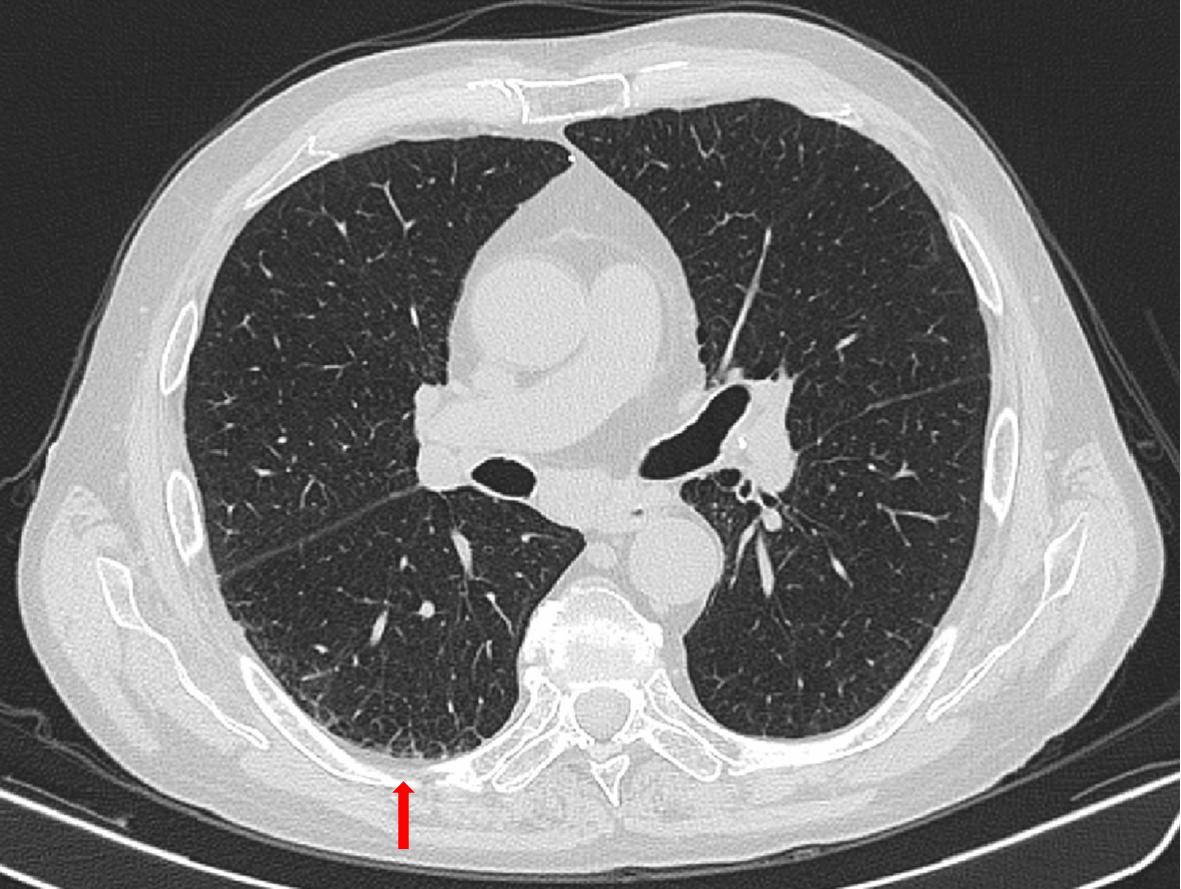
Thoracic HRCT. Pleural thickening and fibrosis
